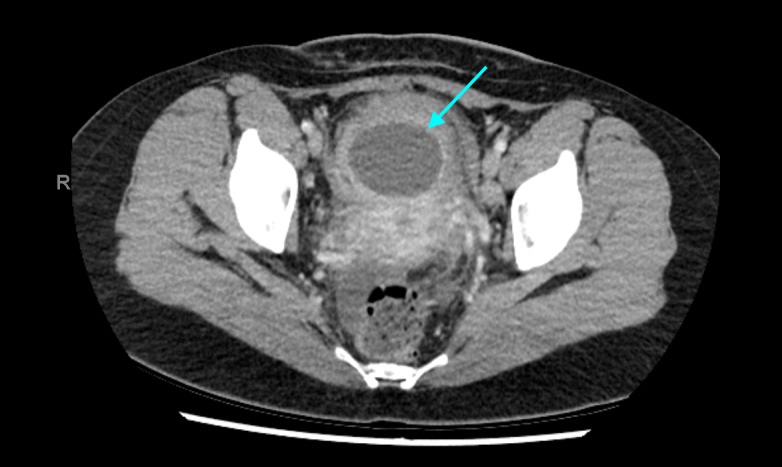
Case 2 Transverse section of CT demonstrating a thick-walled collection between the uterus and the bladder.
